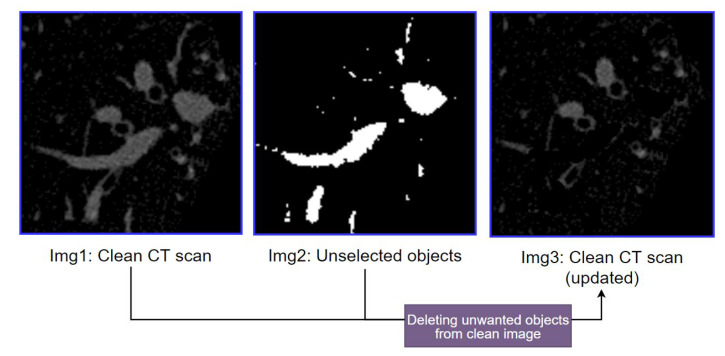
Discarding unwanted bright objects from the clean CT scan.
